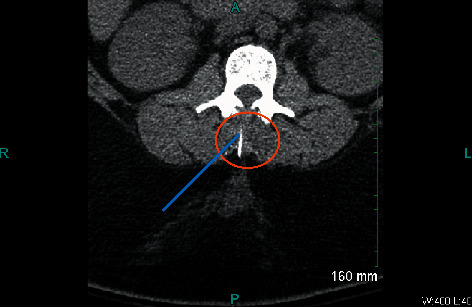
Computed tomography of lumbar spine.
